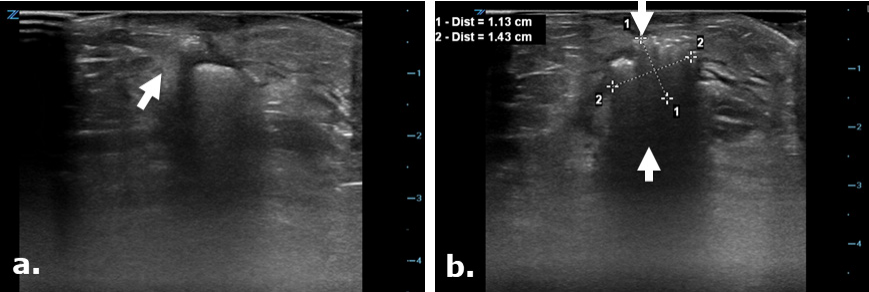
Surgicel in the subcutaneous tissue mimicking a gas containing collection on ultrasound. Focused ultrasound exam images (a, b) were used to evaluate the surgical cut down site following angioplasty in a 75-year-old male. Images depict a focal echogenic mass-like area with posterior acoustic shadowing in keeping with the known Surgicel that was retained post-operatively for hemostasis. This appearance closely mimics an air containing collection.
